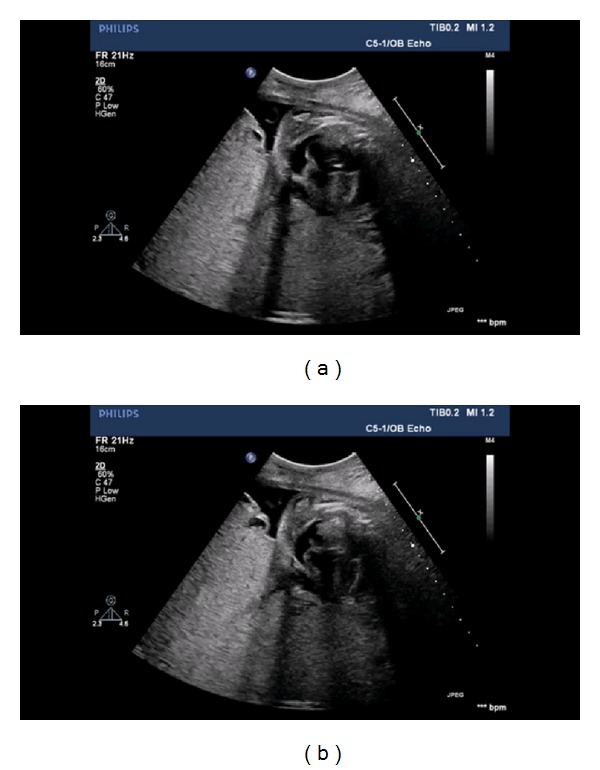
Fetal echocardiographic appearance of dilated coronary sinus.
